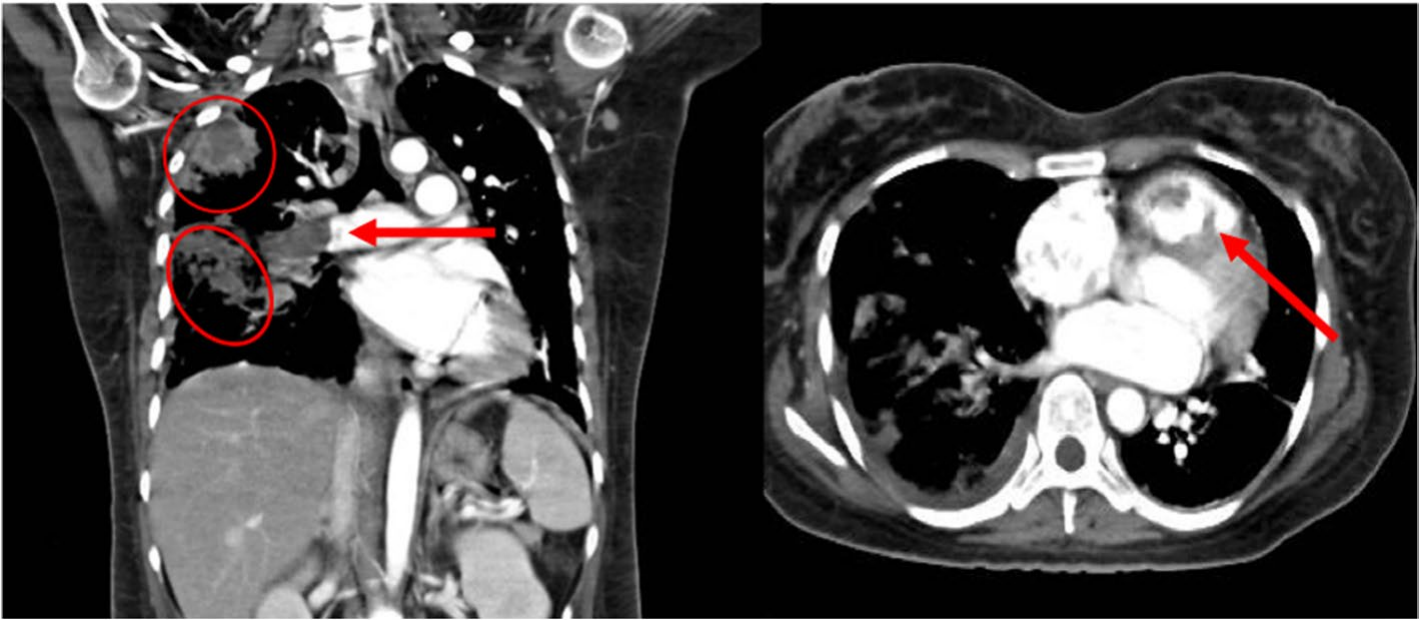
Preoperative enhanced computed tomography on admission showing vegetations in the right ventricle and right PA and abscesses in the right lung
